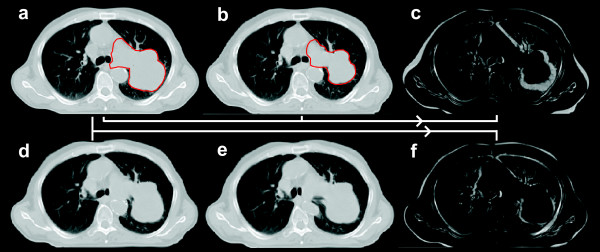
Patient # 12: a) planning CT; b) follow-up CT at the end of treatment; c) difference image between planning CT and follow-up CT; d) deformed follow-up CT using all target and organs-at risk meshes; e) deformed follow-up CT using target, lung and spinal cord; f) difference image between planning CT and deformed follow-up CT based on all target organs-at risk meshes. The contours of the macroscopic primary tumor in the planning CT and the follow-up CT are shown in red. Note the distortion of the vertebral body and the aorta without using meshes of these organs for deformable registration in e).
